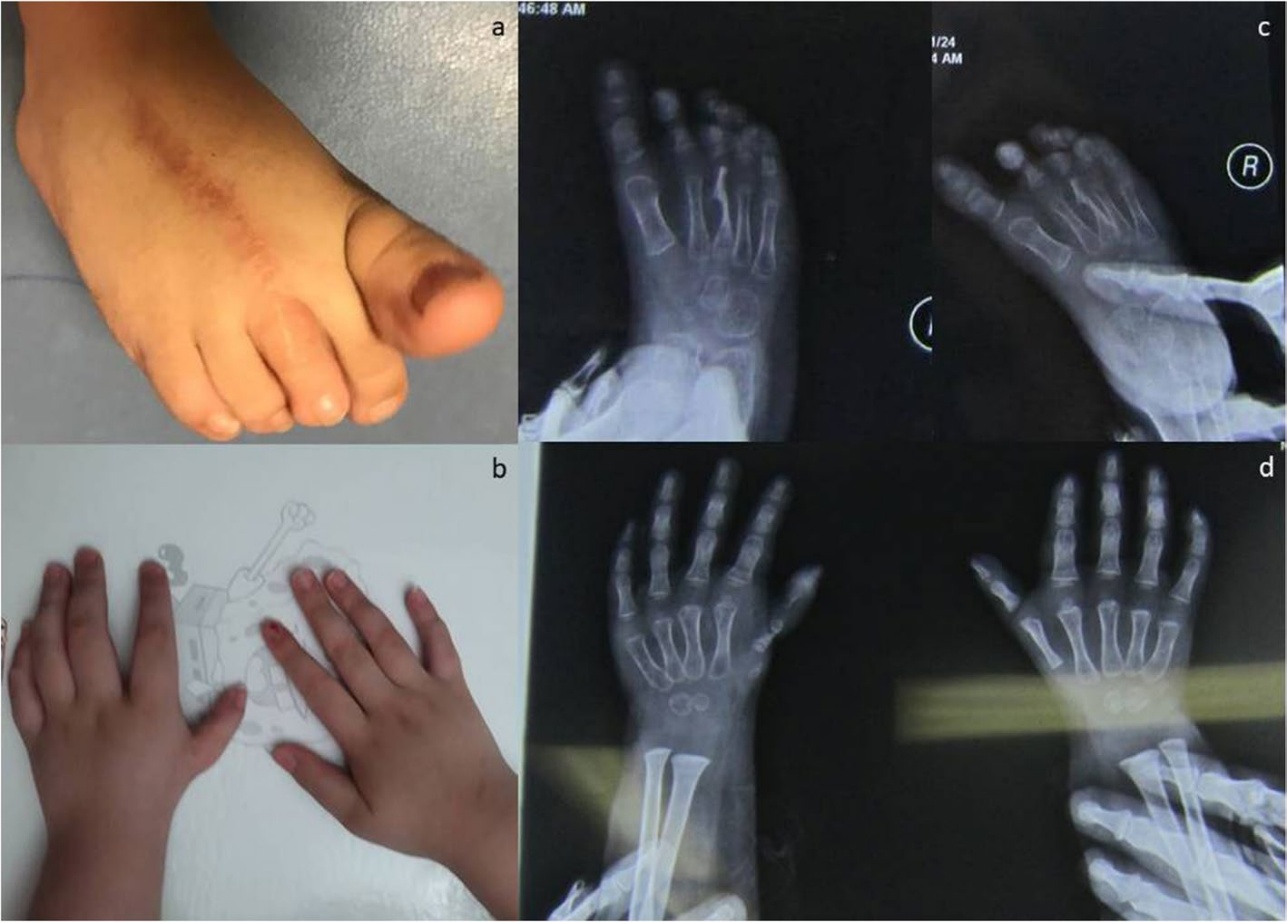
Postoperative appearance of donor site (a), reconstructed hand (b), and a 5-year postoperative X-ray (c)
